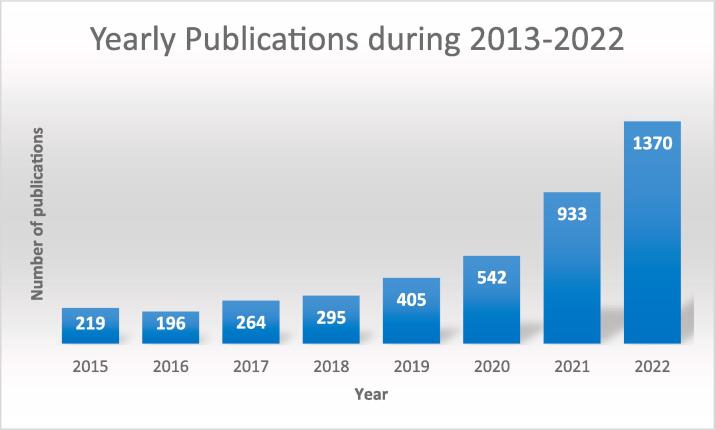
Google Scholar research on publications containing “sonochemistry”, “ultrasound” and “continuous” words.
